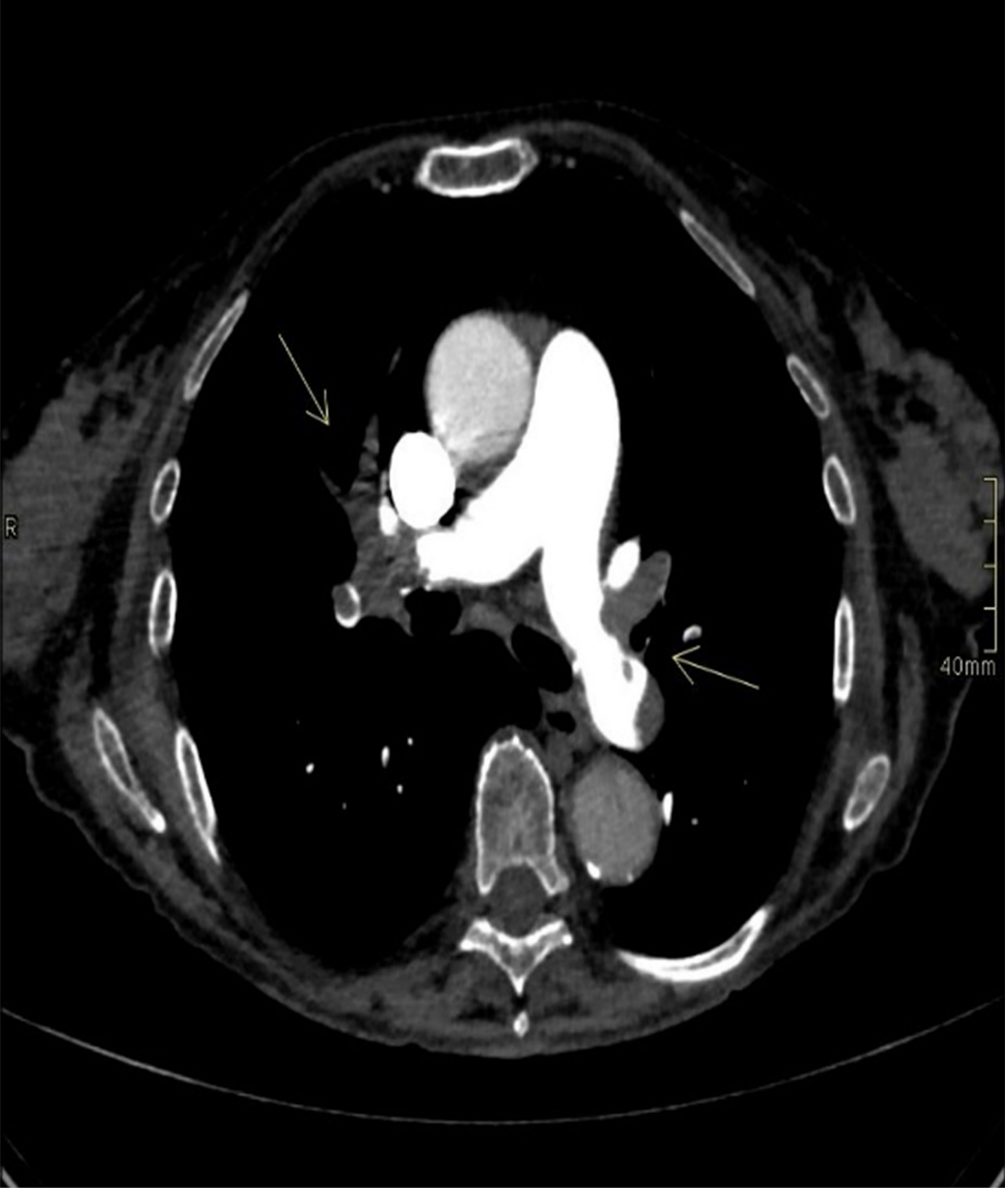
Chest CT scan: the arrows show bilateral lobar and segmental pulmonary embolism.
